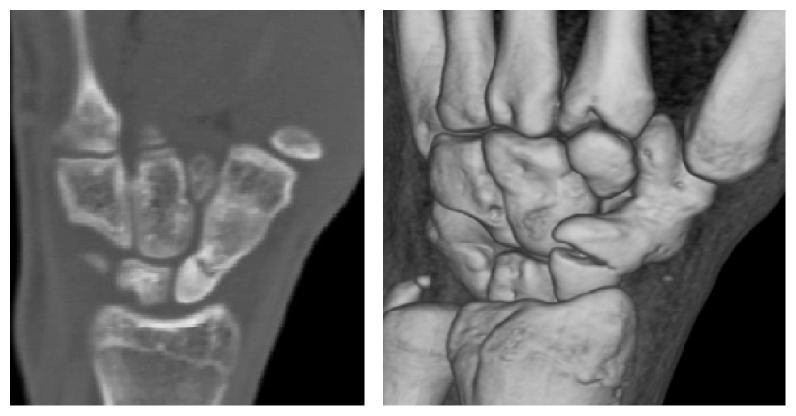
CT scan shows complete Scaphotrapezial synostosis with scaphoid waist fracture.
